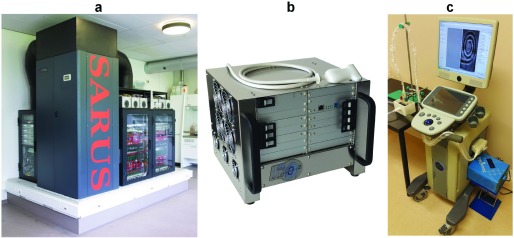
Photographs of three different ultrasound OPs. (a) SARUS developed at the Technical University of Denmark [20], [50]. (b) ULA-OP 256 developed at the University of Florence [21]. (c) Commercially available SonixTouch research scanner with channel domain data acquisition capabilities [61].
